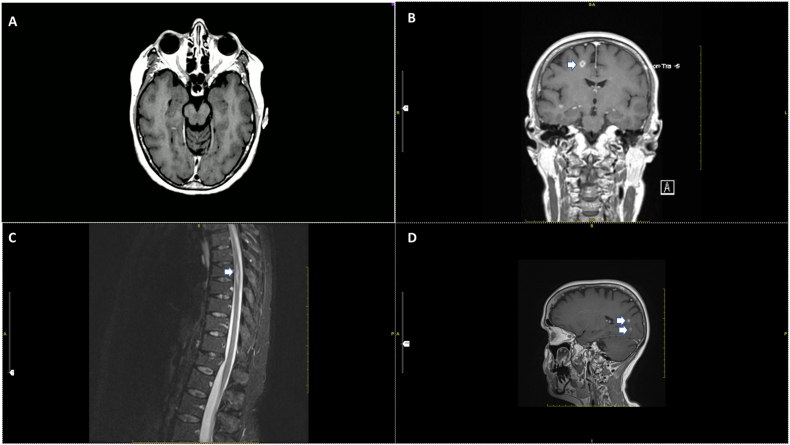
MRI of the brain (a–b) showing multiple post-Gadolinium T_1_-weighted (T1w) hyperintense lesions, some with “open ring” pattern on a a) Post-Gadolinium-T1w sequence of the brain in the axial plane showing bilateral optic nerves enhancement; b) coronal plane;. MRI of the spinal cord on a T_2_-weighted sequence showing an hyperintense spindle D7-D8 lesion.d) sagittal plane.
